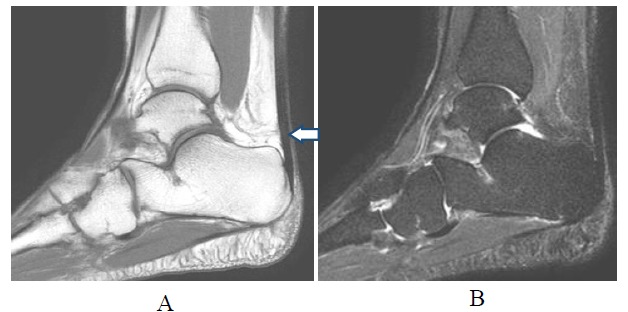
Normal Achilles tendon. T1-weighted and fat suppressed T2-weighted sagittal images show normal hypointense tendon. Small area of internal signal in its distal segment (arrow) may be related to magic angle phenomenon on short TE sequence.
